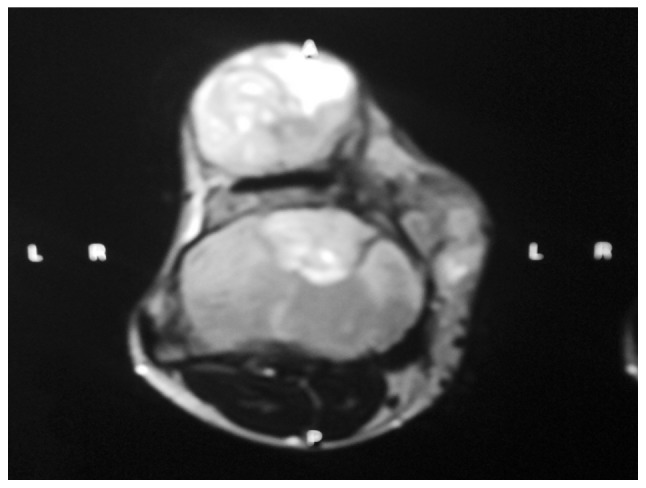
Magnetic resonance image showing the axial section incorporating the anteromedial portion of the tibia.
